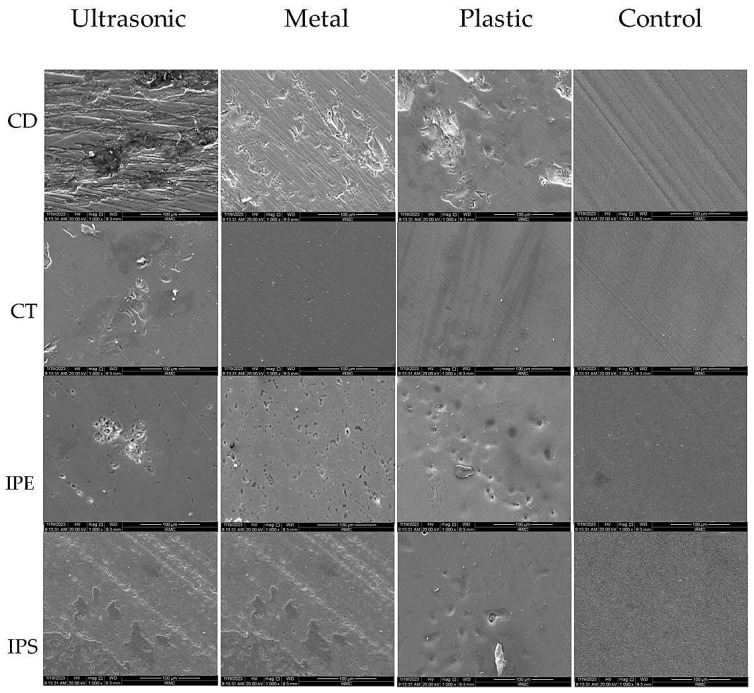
SEM images of the CAD/CAM ceramic materials (CD, CT, IPE, and IPS) treated with three treatment methods: ultrasound, manual metal, and plastic tips in addition to no scaling (control) in ×1000 magnification and scale bar 100 µm. CD; zirconia-reinforced lithium silicate (Celtra Duo). CT; advanced lithium disilicate (CEREC Tessera). IPE; lithium disilicate (IPS Emax CAD). IPS; leucite-reinforced (IPS Empress CAD).
